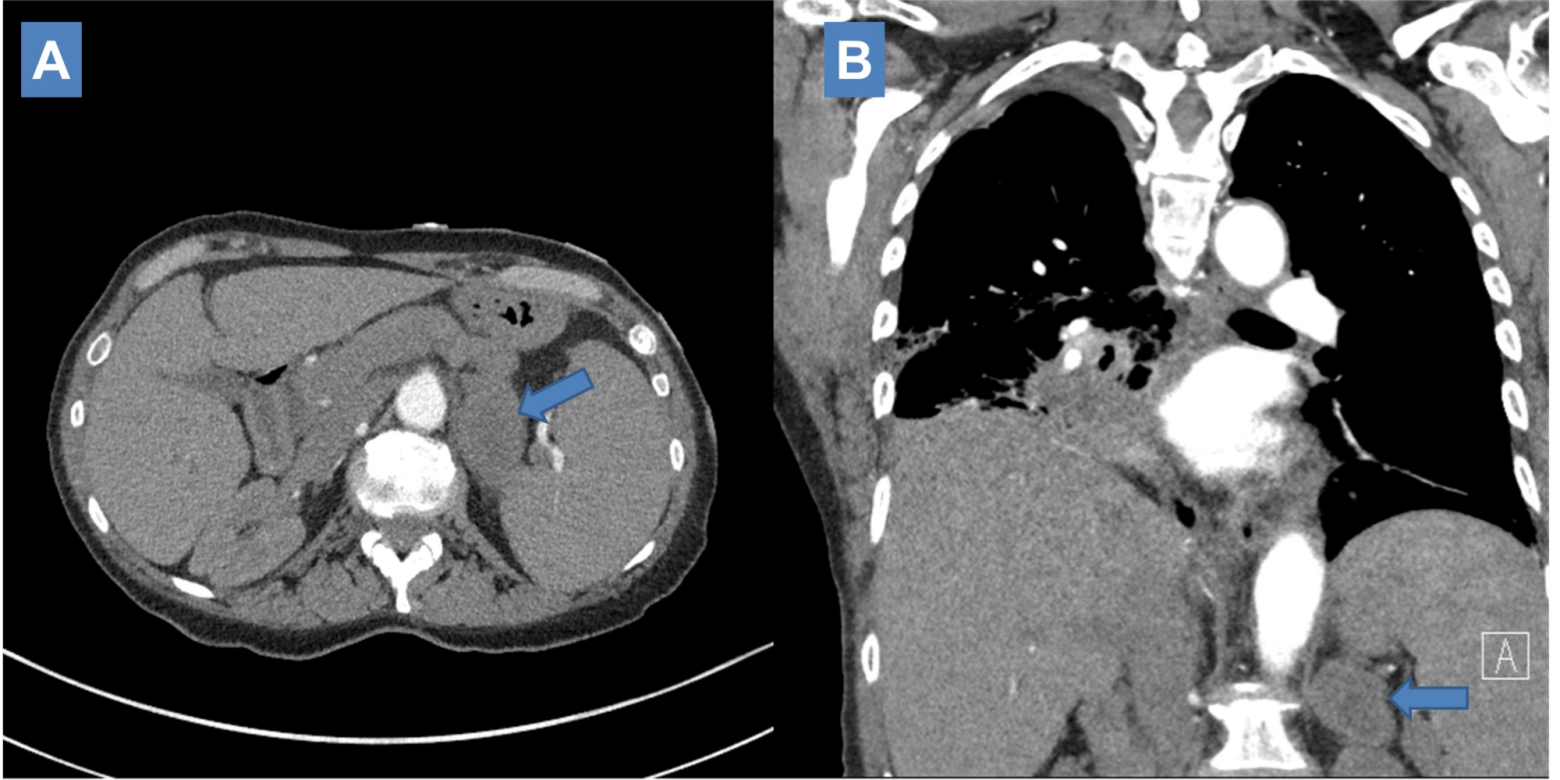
Six-week post definitive treatment computed tomography (CT) scan measuring enlarging left adrenal metastasis (3 cm x 4 cm
